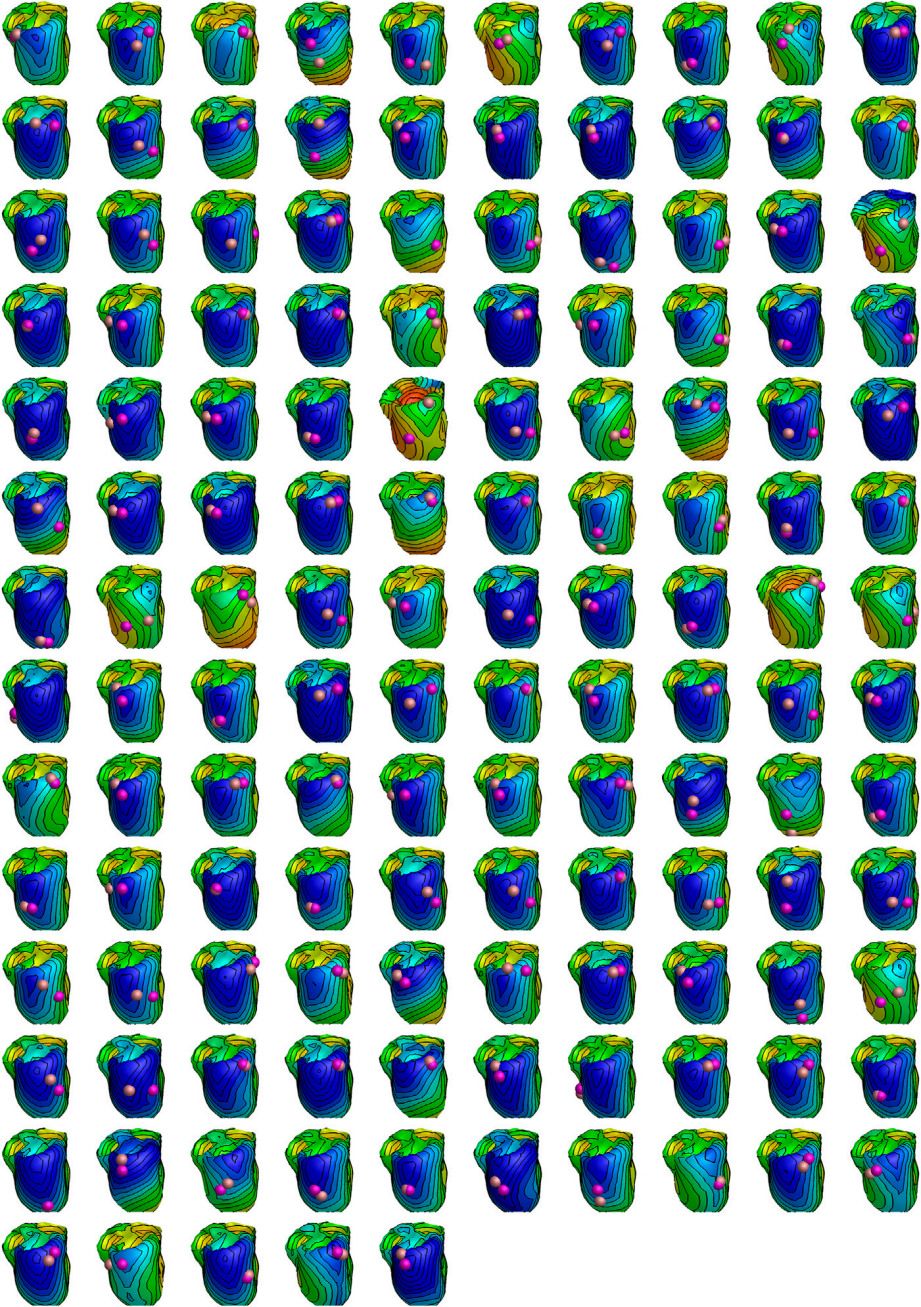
Estimated activation patterns for all 135 patients in the study. All hearts are oriented similarly independent of LV pacing site. Early to late activation is coded with red (earliest) over yellow and green to blue (latest) colors. The coloring is normalized to the patient specific QRS duration. The purple sphere shows the LV pacing site determined by physician by cardiac computed tomography (always in the center of a segment), while the bronze-colored sphere shows the nearest site activating at the measured QLV time. Both spheres are drawn with a diameter of 8 mm.
